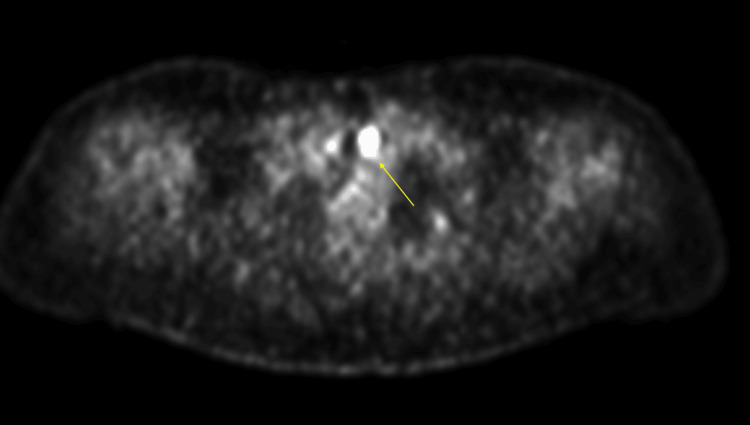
PET scan demonstrating FDG uptake in the left thyroid lobe, as indicated by a yellow arrow FDG: fluorodeoxyglucose; PET: positron emission tomography
